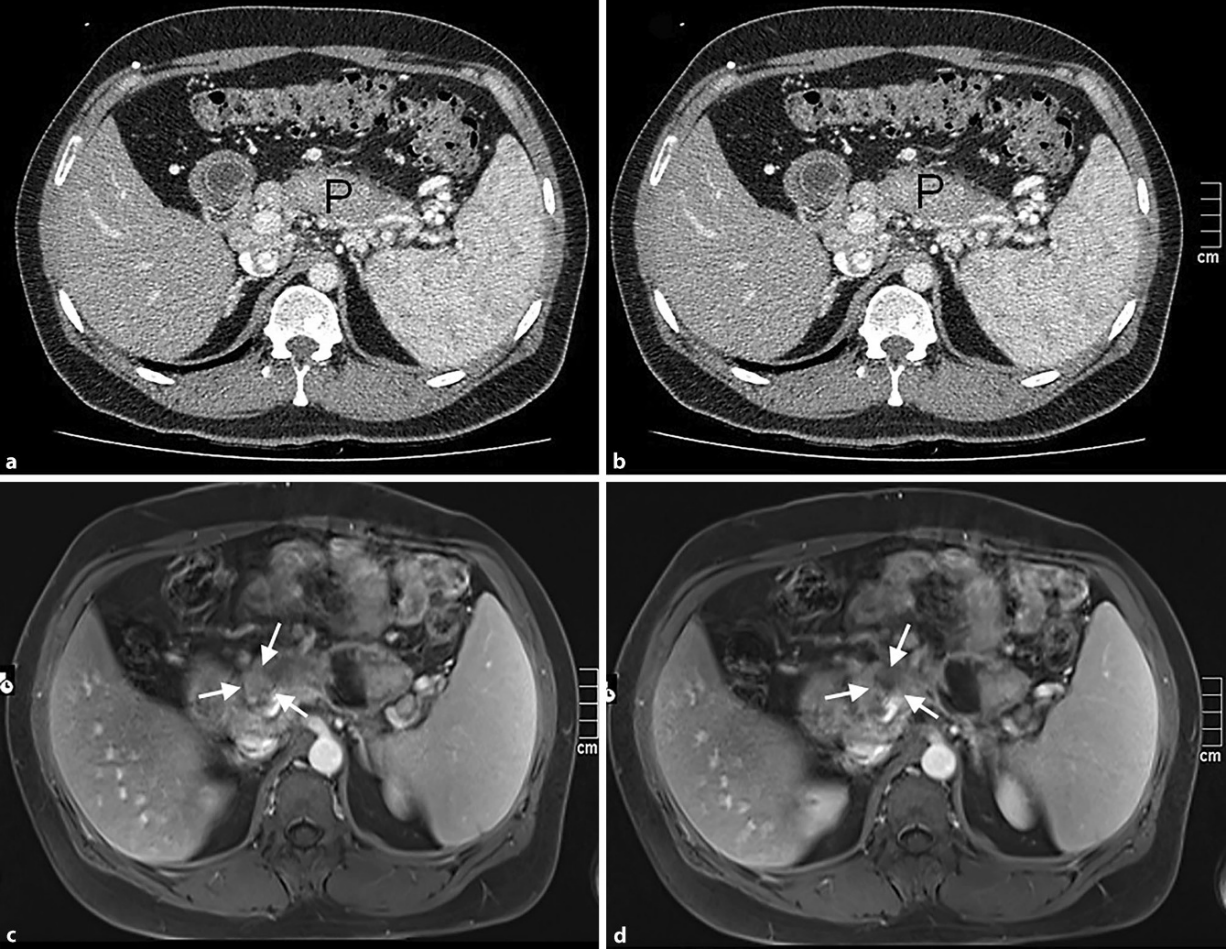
a,b Contrast-enhanced CT showing diffuse enlargement of the body of the pancreas (*P*) with loss of lobular structure. c,d T1-weighted fat-saturated contrast-enhanced magnetic resonance images showing an ill-defined hyopodense lesion (*arrows*) in the head of the pancreas. These findings were interpreted as being consistent with a carcinoma (or cystadenocarcinoma) of the head of the pancreas with diffuse infiltration of the body of the pancreas
